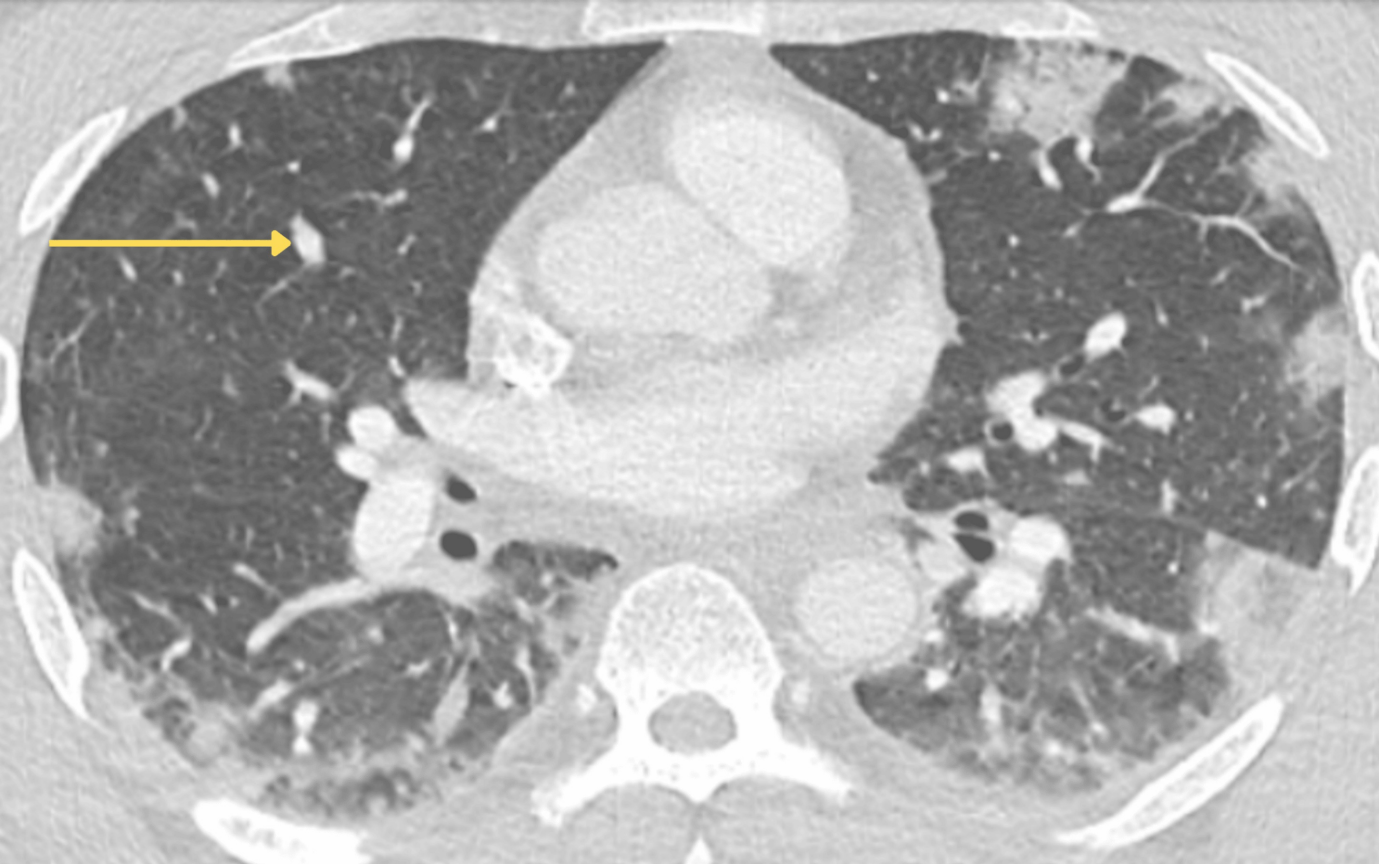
CTA chest on hospital day two showed multiple ill-defined peripheral nodules concerning septic emboli and bilateral ground glass opacities CTA: computed tomography angiography
